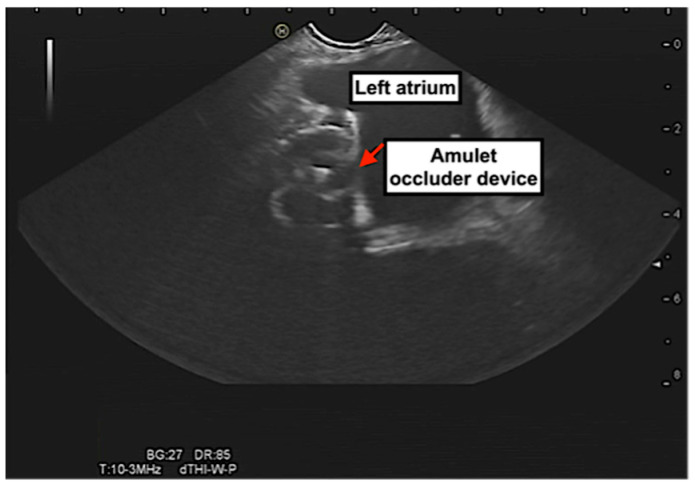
Endoscopic ultrasound demonstrated the morphology of amulet occluder device of left atrial appendage without leak, thrombus or vegetation.
